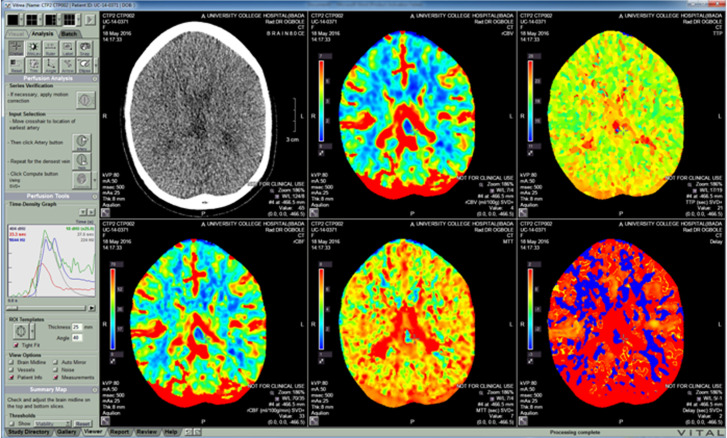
computed tomography perfusion maps on the Vitrea workstation
